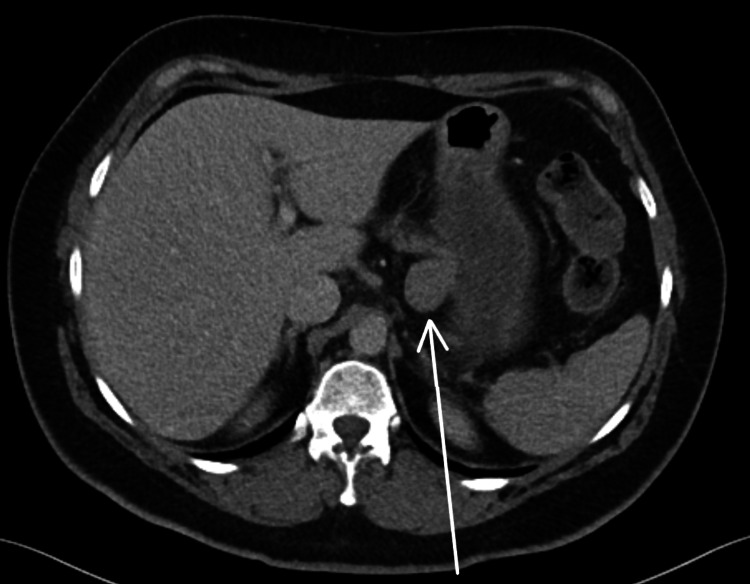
CT scan of abdomen and pelvis. Arrow pointing to the lesion of suspicion.
